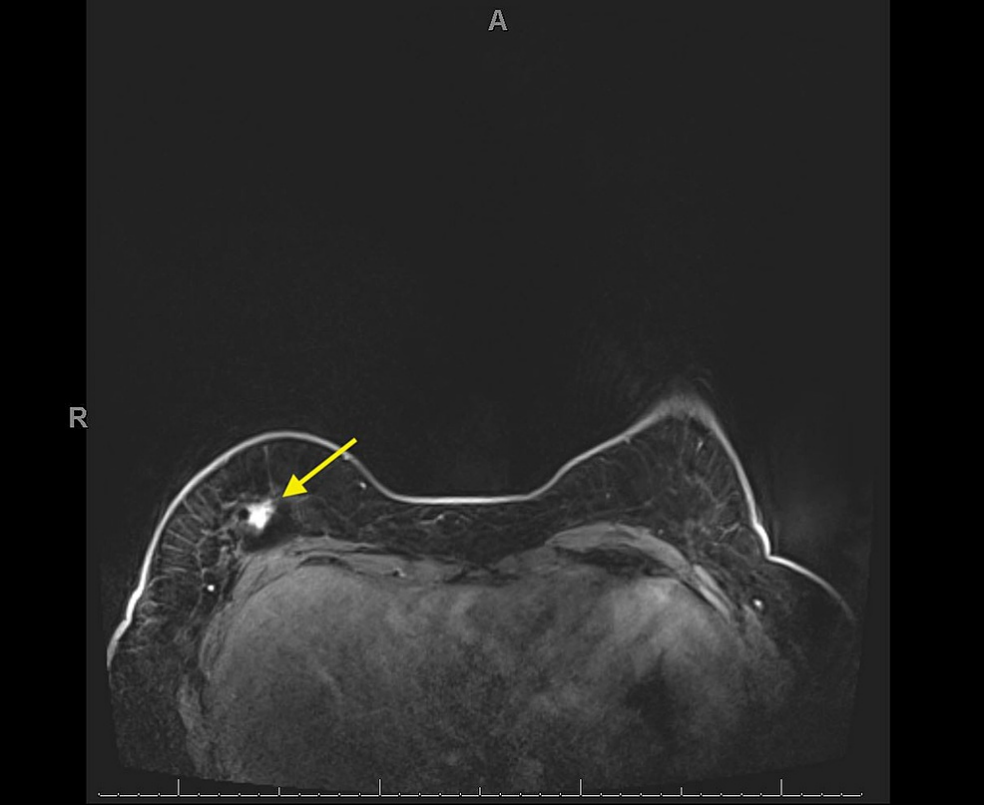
Bilateral breast MRI Right breast irregular enhancing mass (yellow arrow) at the 6:00 axis posteriorly measuring 2.9 cm anterior-posterior by 2.1 cm transverse by 0.9 cm craniocaudal located 8.4 cm from the nipple.
